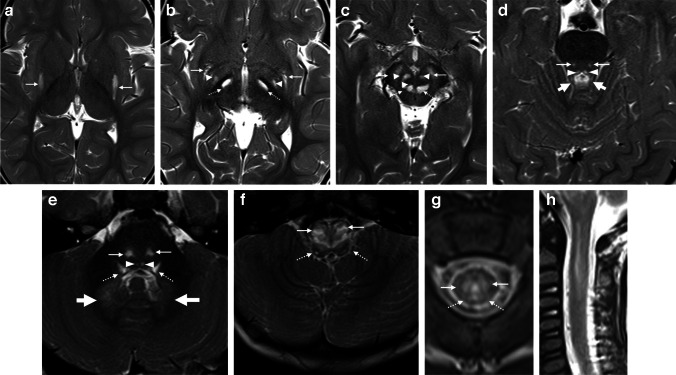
T2-weighted magnetic resonance images through the brain demonstrate features of Leigh syndrome in a 23-month-old boy with confirmed *SURF1* variants. a–c Axial images demonstrate T2 hyperintensity in the bilateral posterior putamina (*arrows* in a), globi pallidi interna (*arrowheads* in b), subthalamic nuclei (*broken arrows* in b), substantia nigra (*arrows* in c), red nuclei (*arrowheads* in c), and periaqueductal grey matter (*broken arrows* in c). d–f Axial images at the level of superior cerebellar peduncles (d), middle cerebellar peduncles (e), and medulla (f) demonstrate hyperintensity within the central tegmental tracts (*arrows* in d and e), medial longitudinal fasciculi (*arrowheads* in d and e), trigeminal sensory nuclei (*broken arrows* in e and f), cerebellar dentate nuclei (*thick arrows* in e), and inferior olivary nuclei (*arrows* in f). g, h Axial (g) and sagittal (h) images demonstrate spinal cord involvement with increased signal in the central cord grey matter (*arrows*) and dorsal columns (*broken arrows*)
